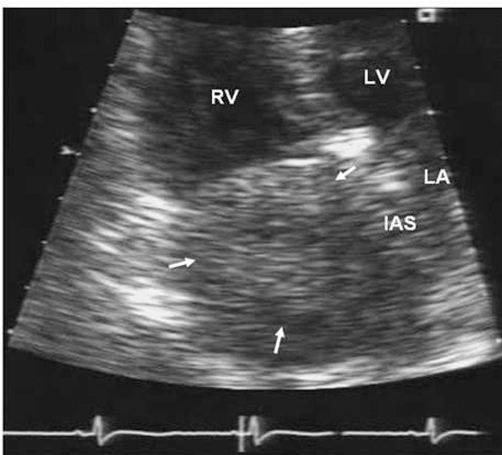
Echocardiographic appearance. Transthoracic echocardiogram apical four chamber view in ventricular diastole shows a 3.5 x 3cm tissue-density echo (arrows) filling the right atrium and reaching the plane of the tricuspid valve annulus. RV, right ventricle. LV, left ventricle. LA, left atrium. IAS, interatrial septum.
